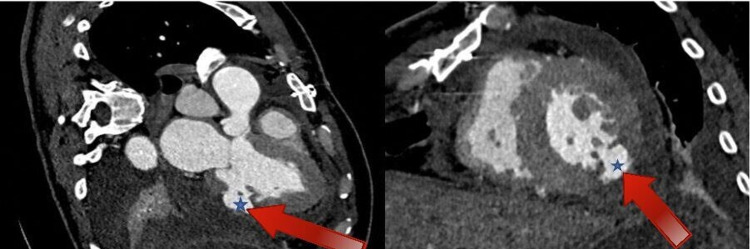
Cardiac CTA showing a contrast-filled outpouch related to the inferolateral wall of the LV associated with a pericardial effusion in keeping with a pseudoaneurysm (red arrow, blue star). CTA, computed tomography angiography; LV, left ventricle
